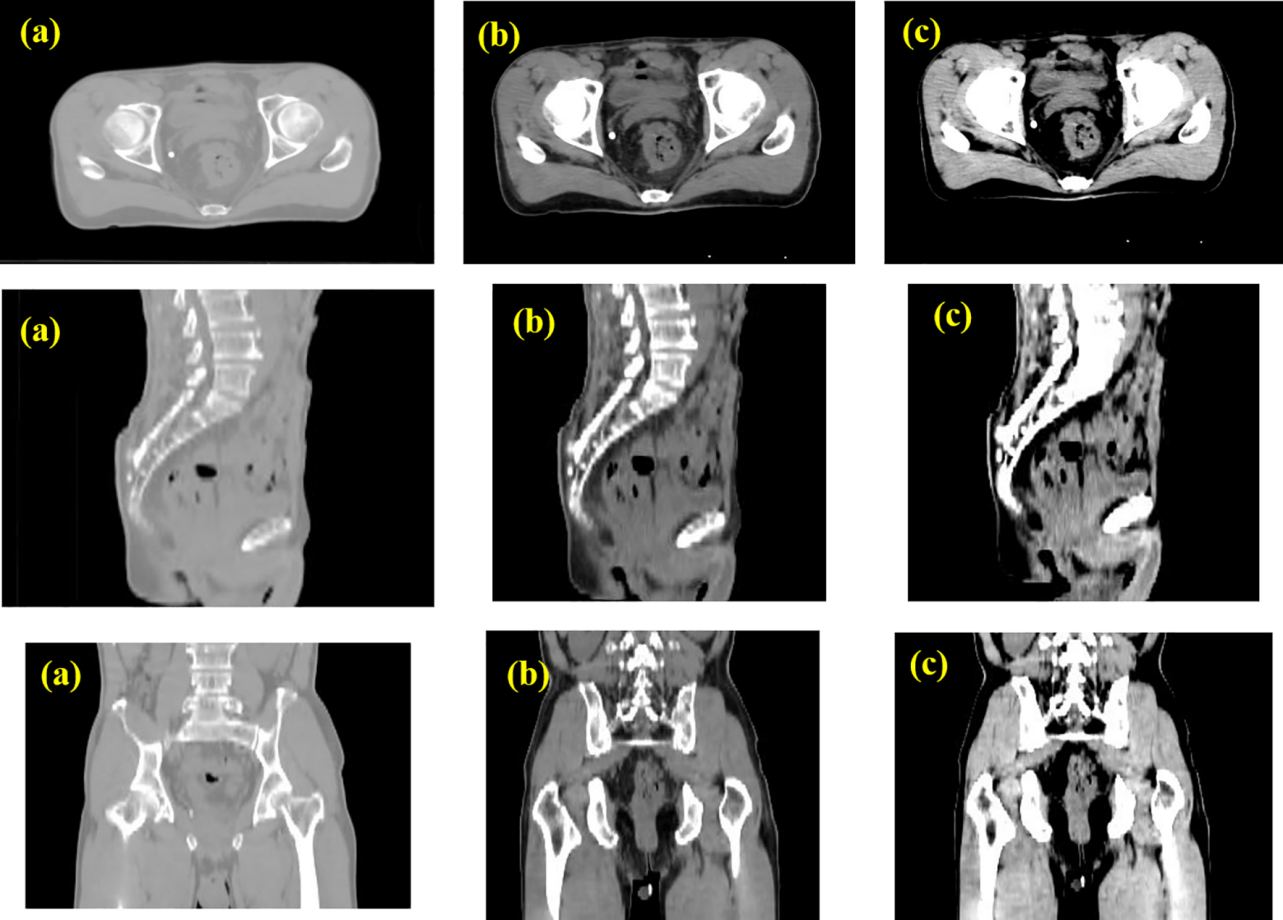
Data pre-processing with CLAHE. (A) Original CT image; (B) CT image intercepted by threshold with HU=(-200~300); (C) CT image transformed by CLAHE.
